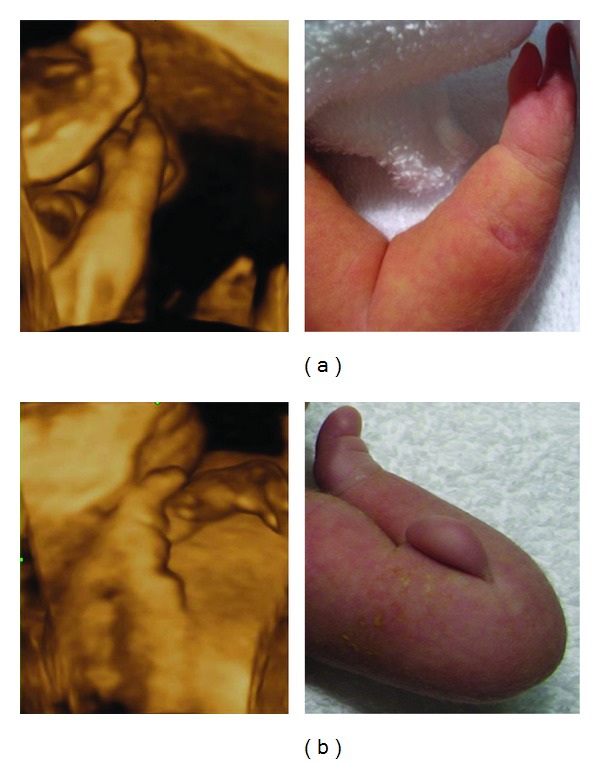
Abnormalities of the forearms in 3D ultrasonography and newborn. (a) Ectrodactylia and (b) monodactylism.
